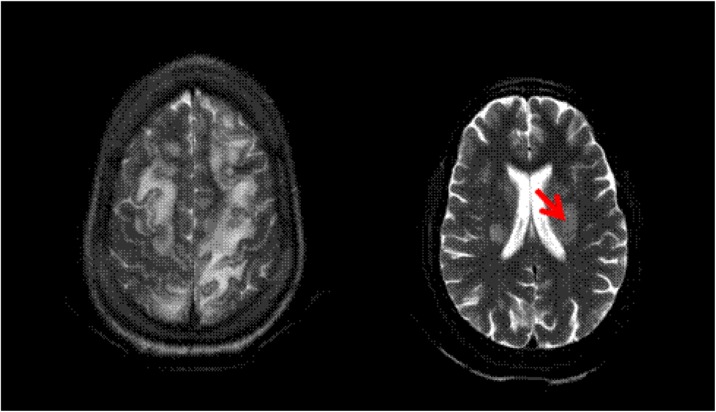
MRI - sites of lesion in PRES
